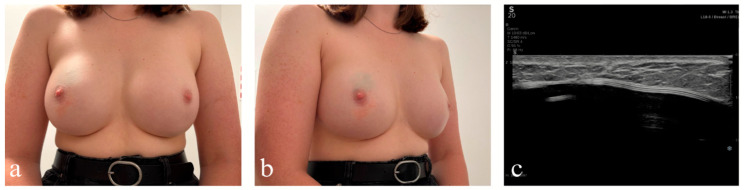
A 24-year-old patient presenting to Group A; (a) front view of the patient’s breasts; (b) side view of the breasts, with the bruising and redness of the right breast more clearly visible (see description in the text); (c) ultrasound image of the bruised area of the right breast, with the implant present (BI-RADS 2).
